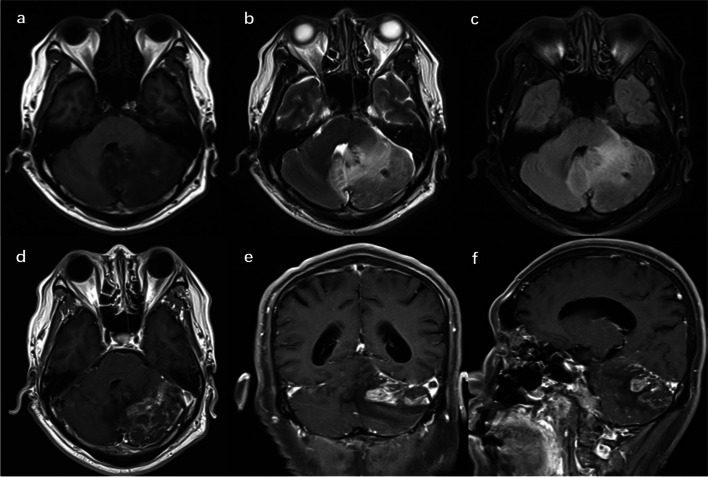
a-c MRI imaging showing an aggravation of the lesion. a Hyperintense on T1WI; b Hyperintense on T2WI; and c FLAIR; d-f Contrast-enhanced imaging showing heterogeneous enhancement of the lesion. d Axial T1WI with gadolinium; e Coronal T1WI with gadolinium and f Sagittal T1WI with gadolinium
